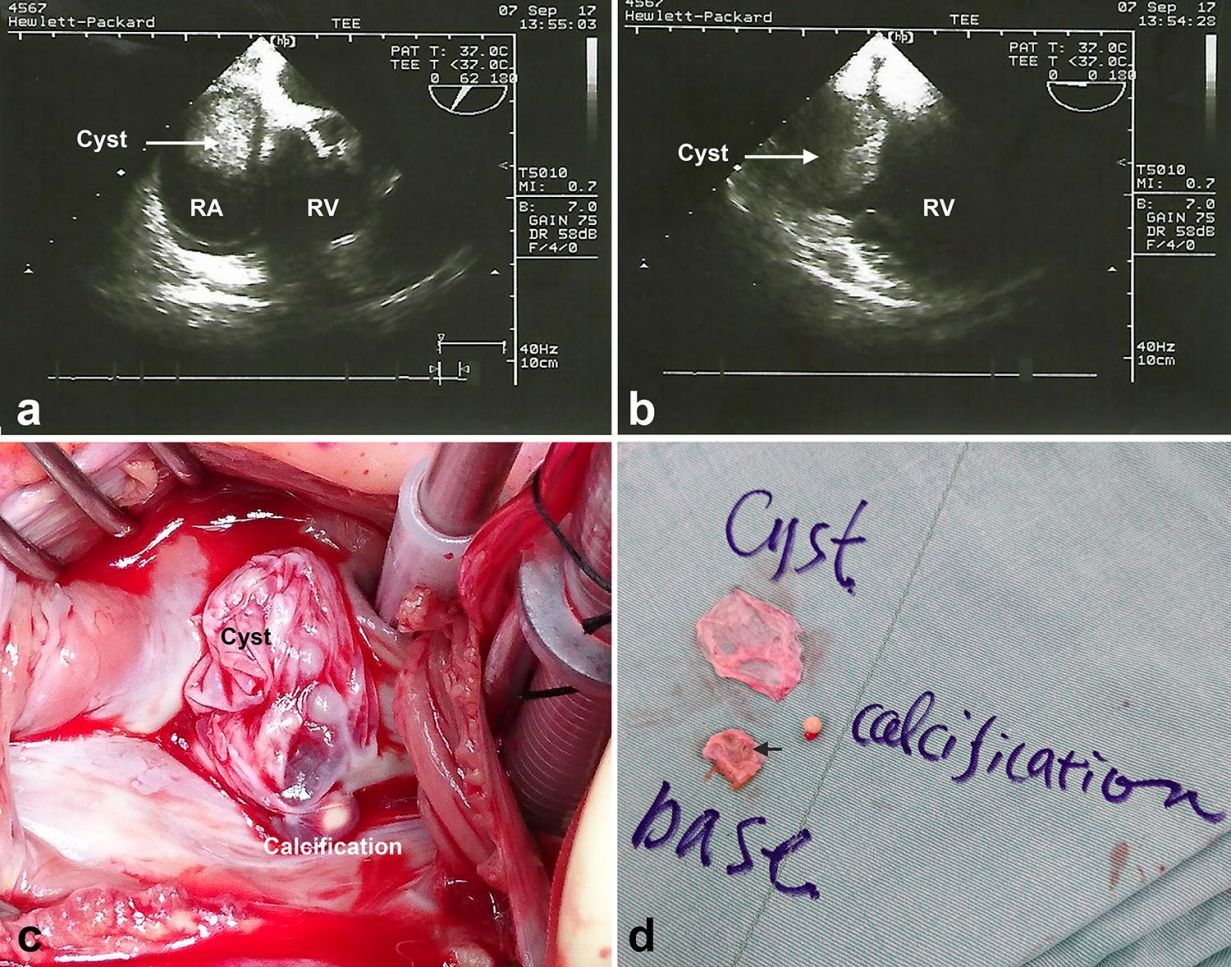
Transthoracic echocardiographic and surgical findings. An oscillating cyst from the septal wall, prolapsing away from the atrioventricular valve in systole (a) and blocking it at the end of diastole (b) (RV: right ventricle; RA: right atrium). The blood cyst originating from the fossa ovalis with a pinhole-sized defect (black arrow) (c, d)
